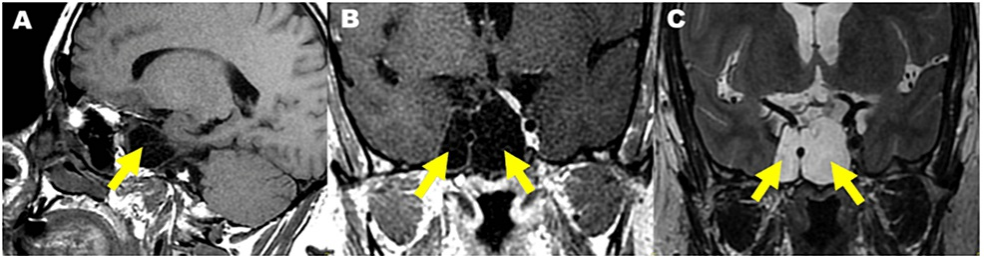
Injected MRI images: sagittal T1 (A), coronal T1 (B), coronal T2 (C): showing a cystic pituitary macroadenoma with a liquid component in T1 hyposignal, T2 hypersignal, without enhancement (Department of Radiology Hassan II University Hospital, Fez).
